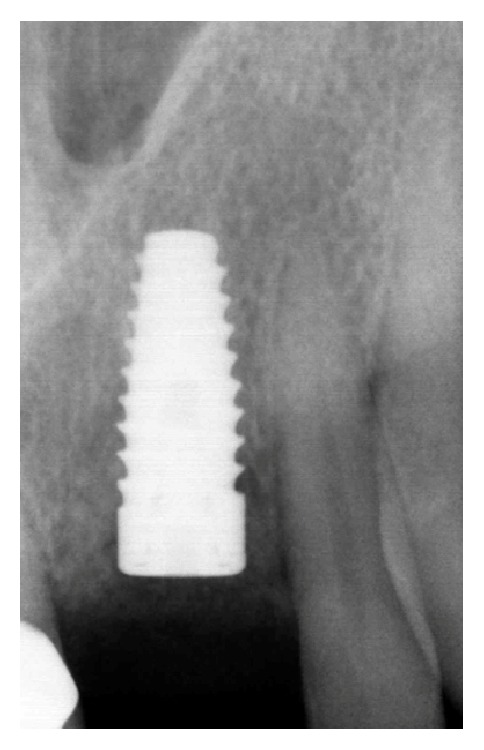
Radiographical control at 2-year follow-up.
